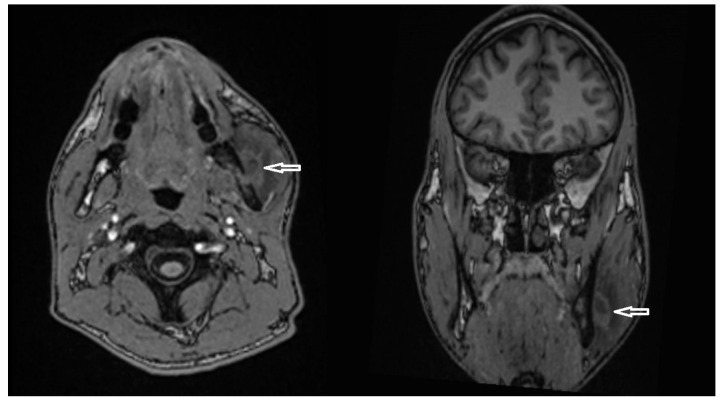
Axial (left) and coronal (right) T1-weighted MRI images showing a hyperintense lesion adjacent to the lateral aspect of the distal mandibular ramus (white arrows), suggestive of a developing abscess.
